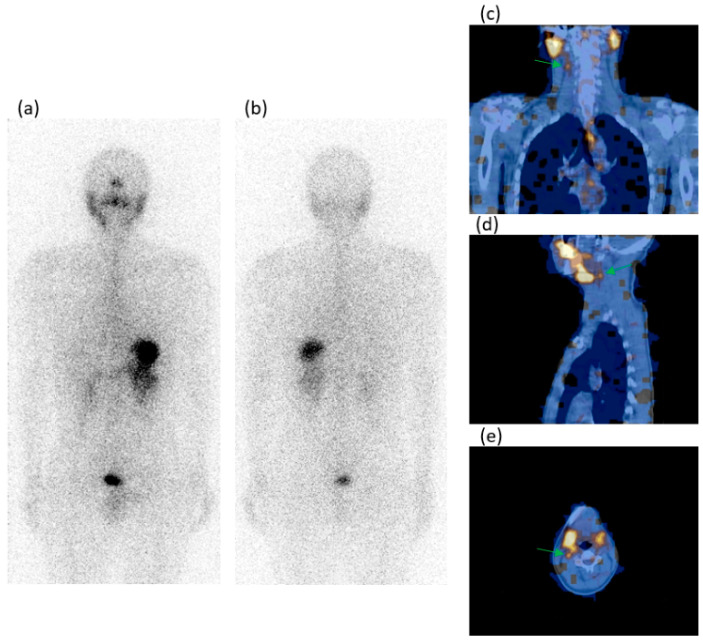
A 28-year-old male patient who underwent a total thyroidectomy and radioiodine ablation for a 15 mm T1b papillary carcinoma (PC) classic variant. At surgery, mETE and neck LN laterocervical metastasis were identified. During the follow-up, the WBS in anterior/posterior (a,b) views did not detect any foci of pathologic radioiodine uptake. The SPECT/CT on the coronal/sagittal/transaxial (c–e) slides evidenced a radioiodine avid focus (green arrows) in the neck (right laterocervical region), which on the sagittal slides was located behind the submandibular salivary gland. This focus was classified as a metastatic lymph node and was treated with a second surgery and radioiodine ablation. Thyroglobulin levels were in hypothyroidism range before the present scintigraphy: 6.6 ng/mL; AbTg: absent.
